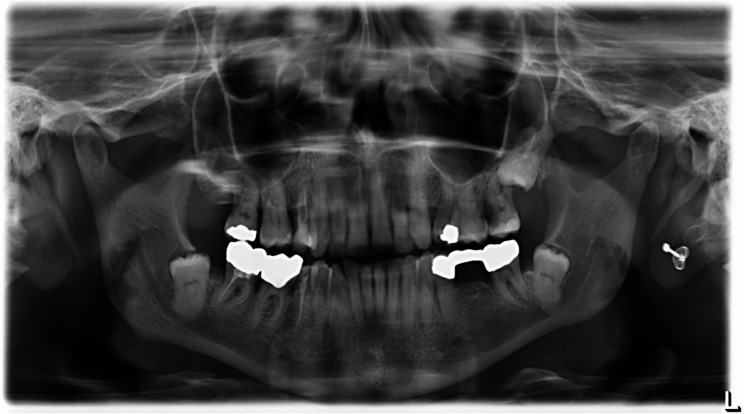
Panoramic radiograph of a patient diagnosed with squamous cell carcinoma localized in the floor of the mouth before recieving radiotherapy, multiple restaurations are visible on the lower molars and on teeth 17 and 25 in metallic opacity. We thank the Oral Surgery Department of Düsseldorf University Hospital for providing these radiographs
